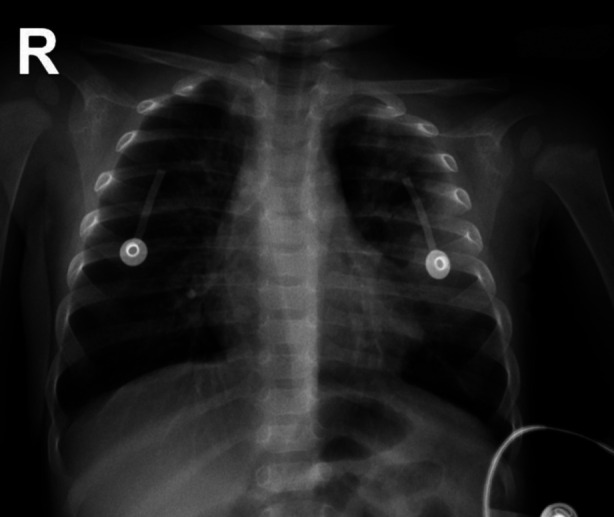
Frontal chest view X-ray showing non-homogeneous opacity in the left upper and middlezone.
